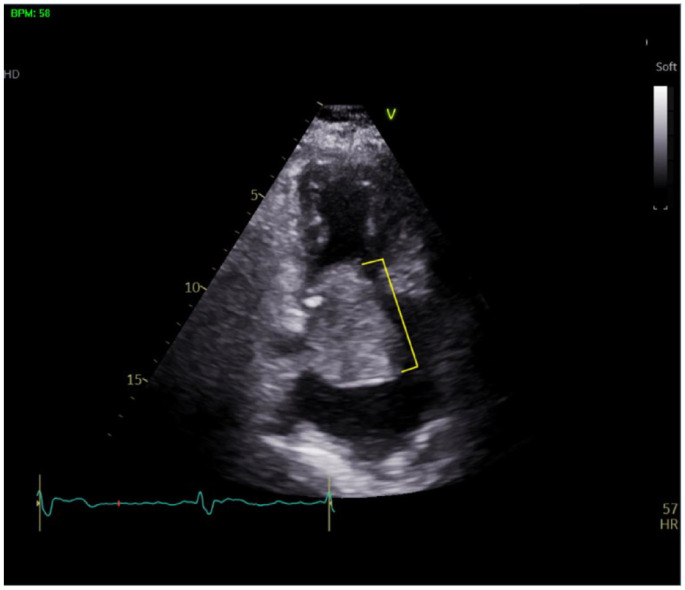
Transthoracic echocardiogram of left atrial myxoma (yellow bracket).
